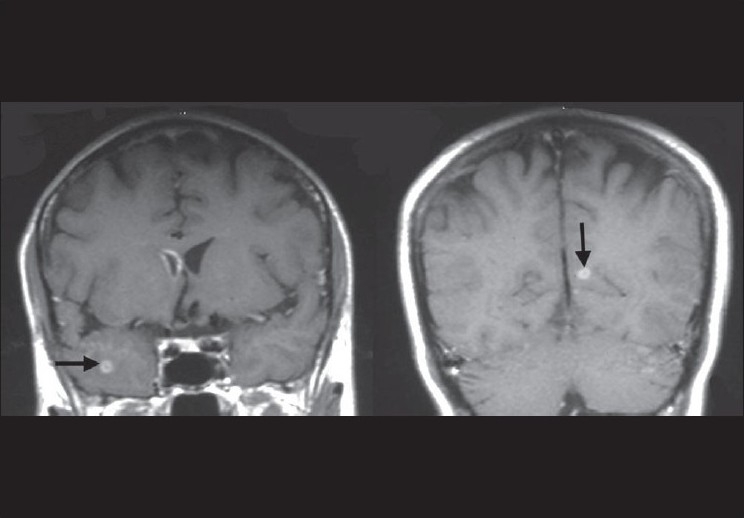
MRI coronal postcontrast T1 sequence shows ringenhancing lesion in the right anterior temporal pole and left parietooccipital region (black arrows); there is enhancement involving the right frontal horn, suggesting ventriculitis
